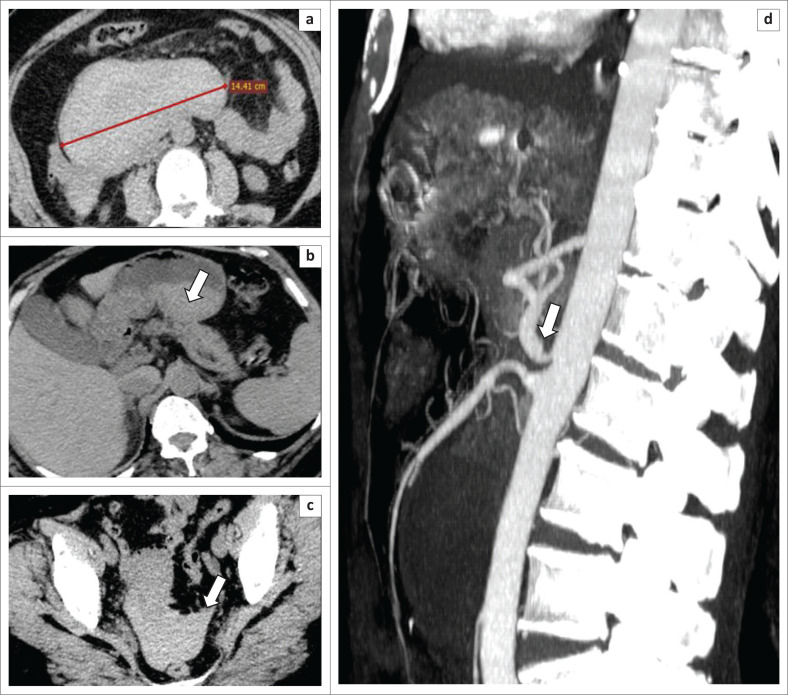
A 64-year-old woman with persistent diffuse abdominal pain, admitted for haematemesis and melena. Axial non-enhanced computed tomography demonstrates a homogeneous hyperdense haematoma of approximately 14 cm (a), haemorrhage within the stomach (arrow, b) and haemoperitoneum (arrow, c). Arterial phase sagittal reconstruction (d) demonstrates moderate coeliac artery compression by the median arcuate ligament with no visible pancreatico-duodenal artery aneurysms.
